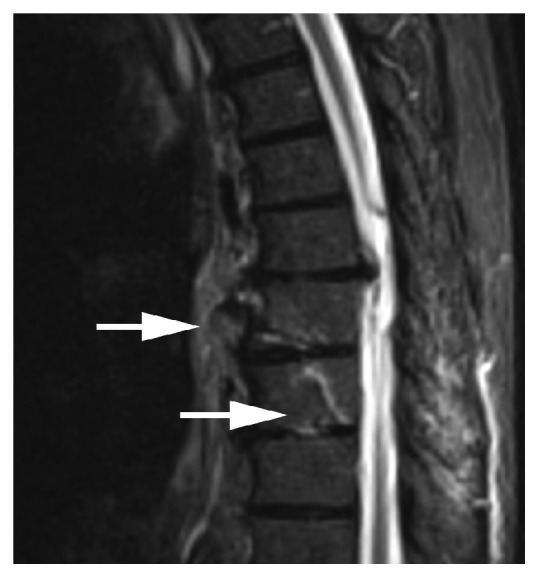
Sagittal MRI, T2 short tau inversion recovery (STIR) sequence, showing unstable acute fractures of the T8-T9 vertebrae (*arrows*).
